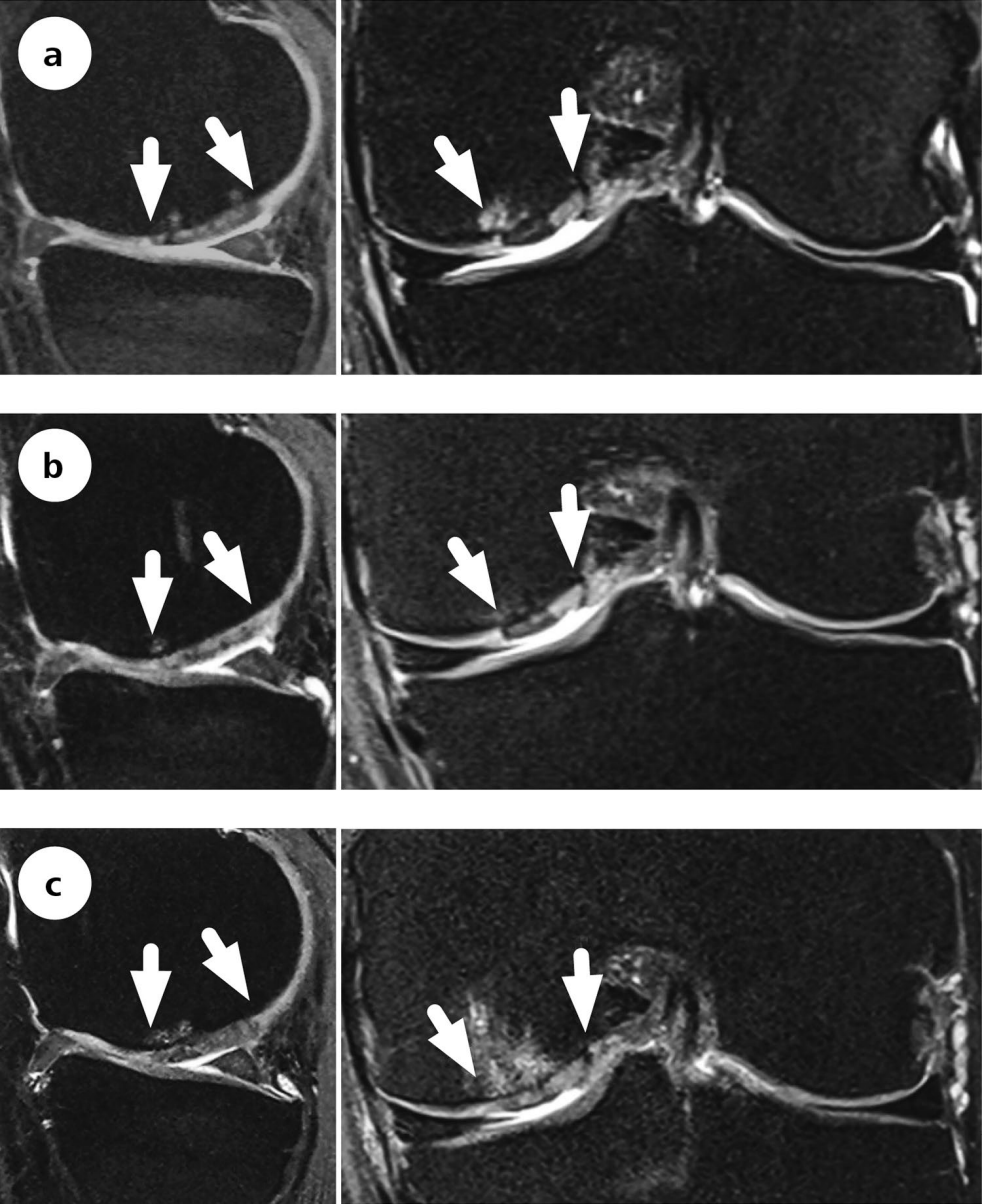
Representative MRI (1.5 T, t2PDw) with images of an AMIC^®^ glued at the medial femoral condyle at 2 years (a), 5 years (b) and 10 years (c). The Cincinnati score was 28/100/90/90 at baseline, 2, 5 and 10 years, respectively. The arrows indicate area of the surgical procedure
